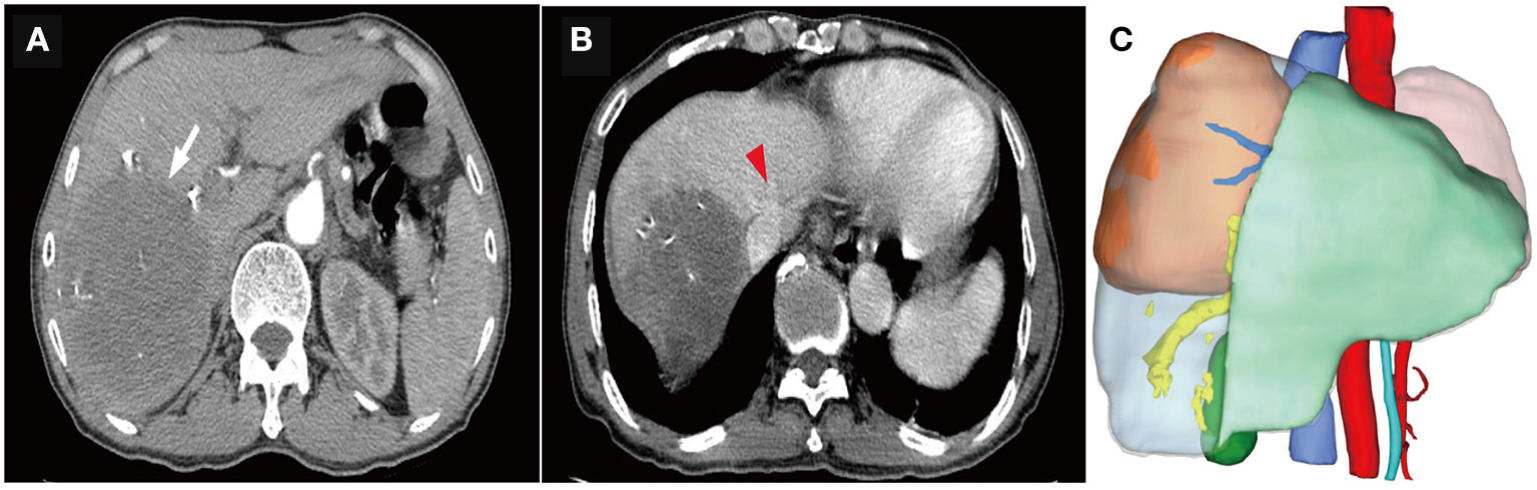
Computed tomography (CT) scans and three-dimensional reconstruction after portal vein embolization (PVE) and multimodality therapy. (A) The tumor remarkably regressed (the white arrow), with complete disappearance of intratumoral enhancement on the artery phase. (B) The distance between the tumor and the middle hepatic vein (the red arrow head) was significantly extended. (C) The ratio of future liver remnant (the light green area) to the standard liver volume (SLV) was 67.74%, which was well-tolerated for hepatectomy.
